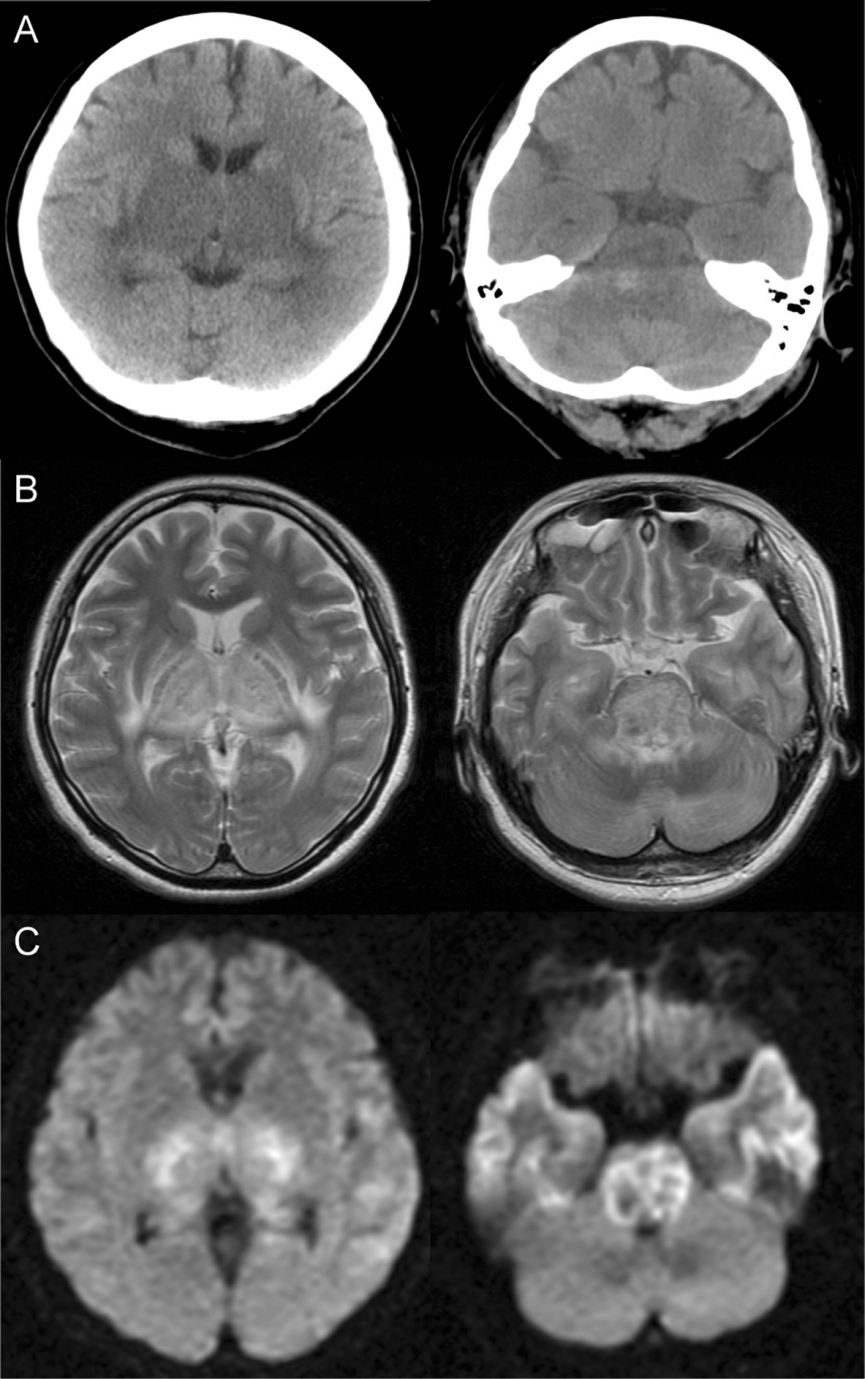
Clinical imaging. (A) CT showed low density area in the bilateral thalami, basal ganglia, medial temporal lobe, and brainstem. The brainstem was swollen, and high density area was observed in the pons. (B) MRI (T2-weighted image) revealed high signal intensity in the bilateral thalami, basal ganglia, medial temporal lobe, and brainstem. (C) MRI (diffusion-weighted image) also showed high signal intensity in the same area.
